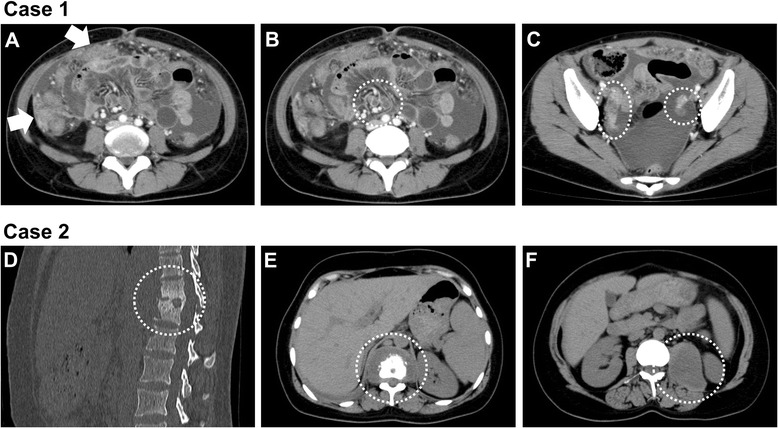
Abdominal CT findings of case 1 (a–c) and case 2 (d–f) at diagnosis. a, Moderate ascites and intraabdominal mass lesions (*arrows*). b, intraabdominal lymph nodes swelling (*in the circle*). c, bilateral adnexal masses (*in the circle*). d and e, Osteosclerotic lesion of Th11-Th12 vertebra with surrounding abscess (*in the circle*). f, A large low attenuation mass in the left psoas muscle (*in the circle*)
